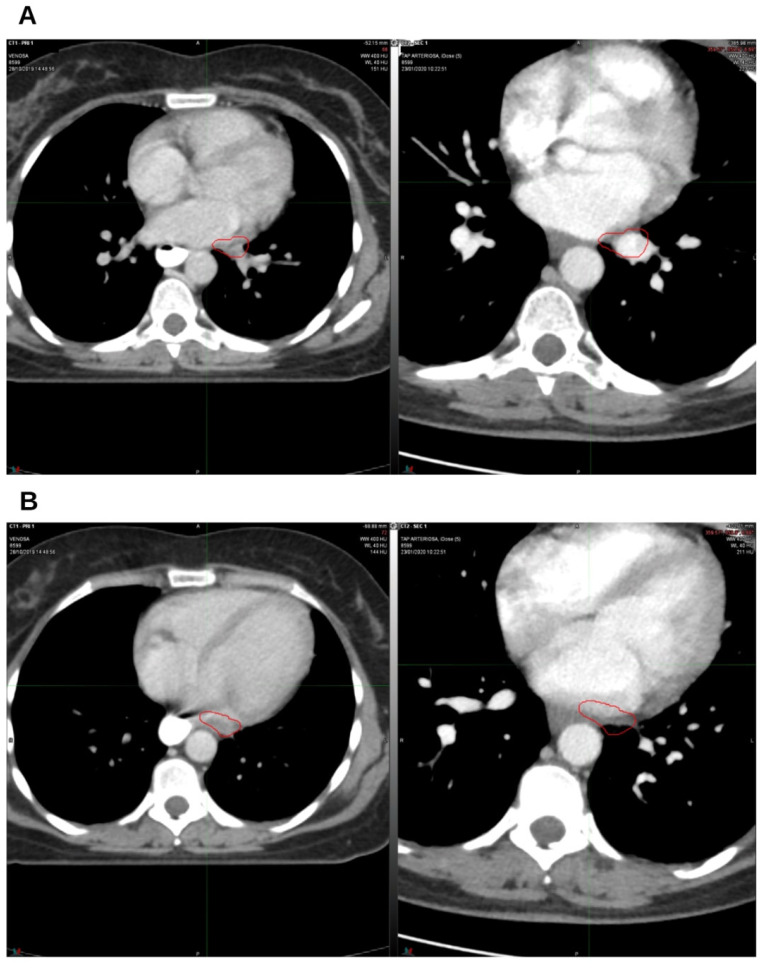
The first follow-up at 3 months off. On the left, CT from October 2019, pre-radiation therapy treatment; on the right, CT from January 2020. (A) Hilar lymph node; (B) left para-aortic lymph node. The indicated region highlights the boundary of the treated target.
